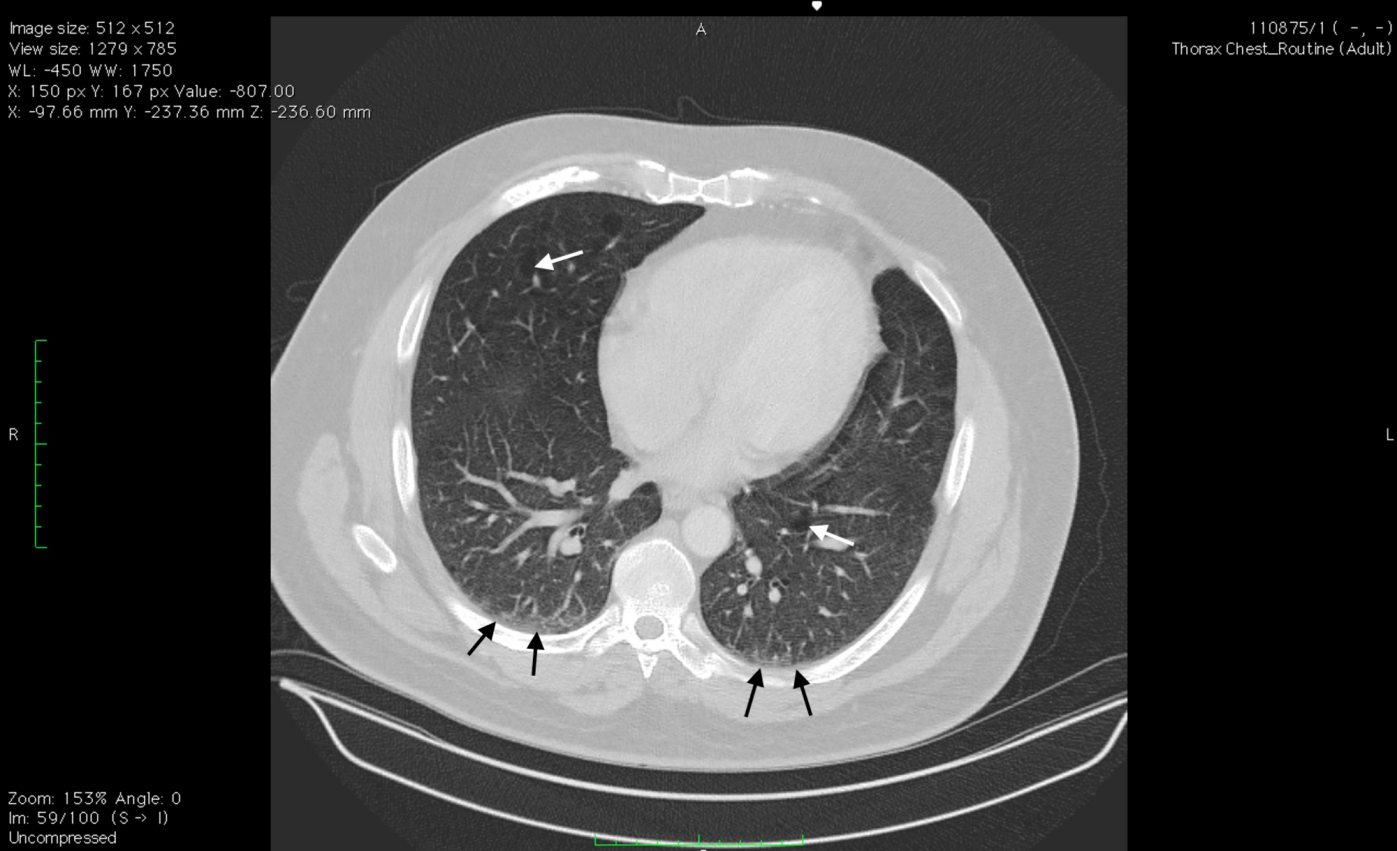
Multidetector Helical Computed Tomography Scan of the Chest, Transverse View This image displays a transverse view of our patient with stable bilateral emphysematous changes shown by the white arrows, as well as bilateral lower lobe dependent atelectasis shown by the black arrows.
